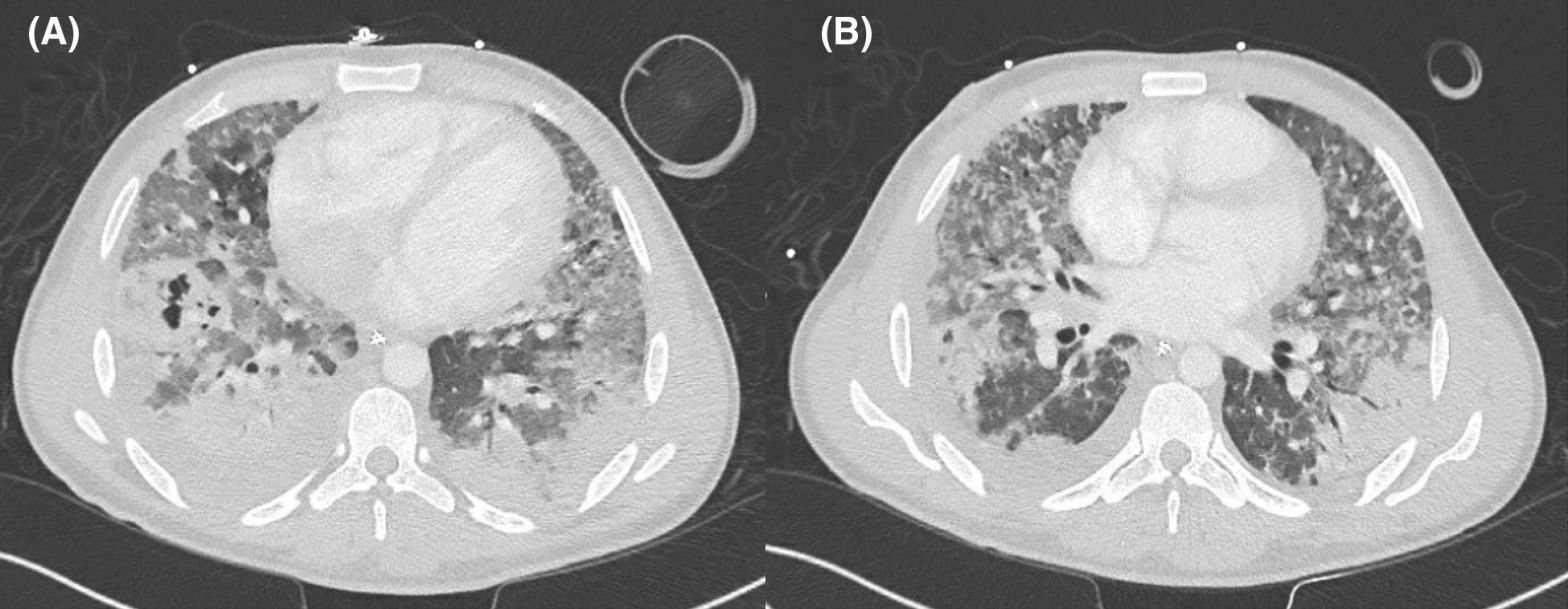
A,B: CT chest, showing diffuse bilateral opacification of both lungs fields with multiple areas of patchy consolidations with air bronchogram and areas of necrosis
